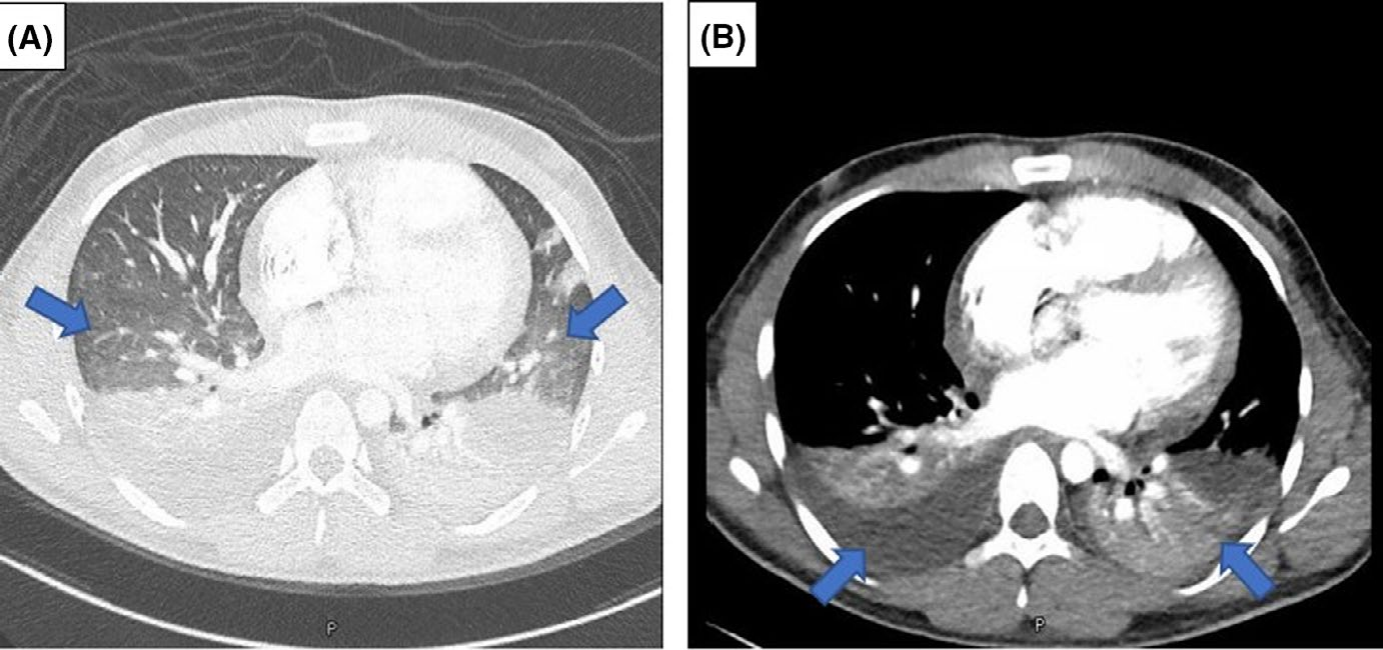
Contrast‐enhanced CT of the thorax. (A) shows bilateral ill‐defined ground‐glass opacities in the lung bases, and (A, B) depicts bilateral pleural effusion and adjacent lung collapse
